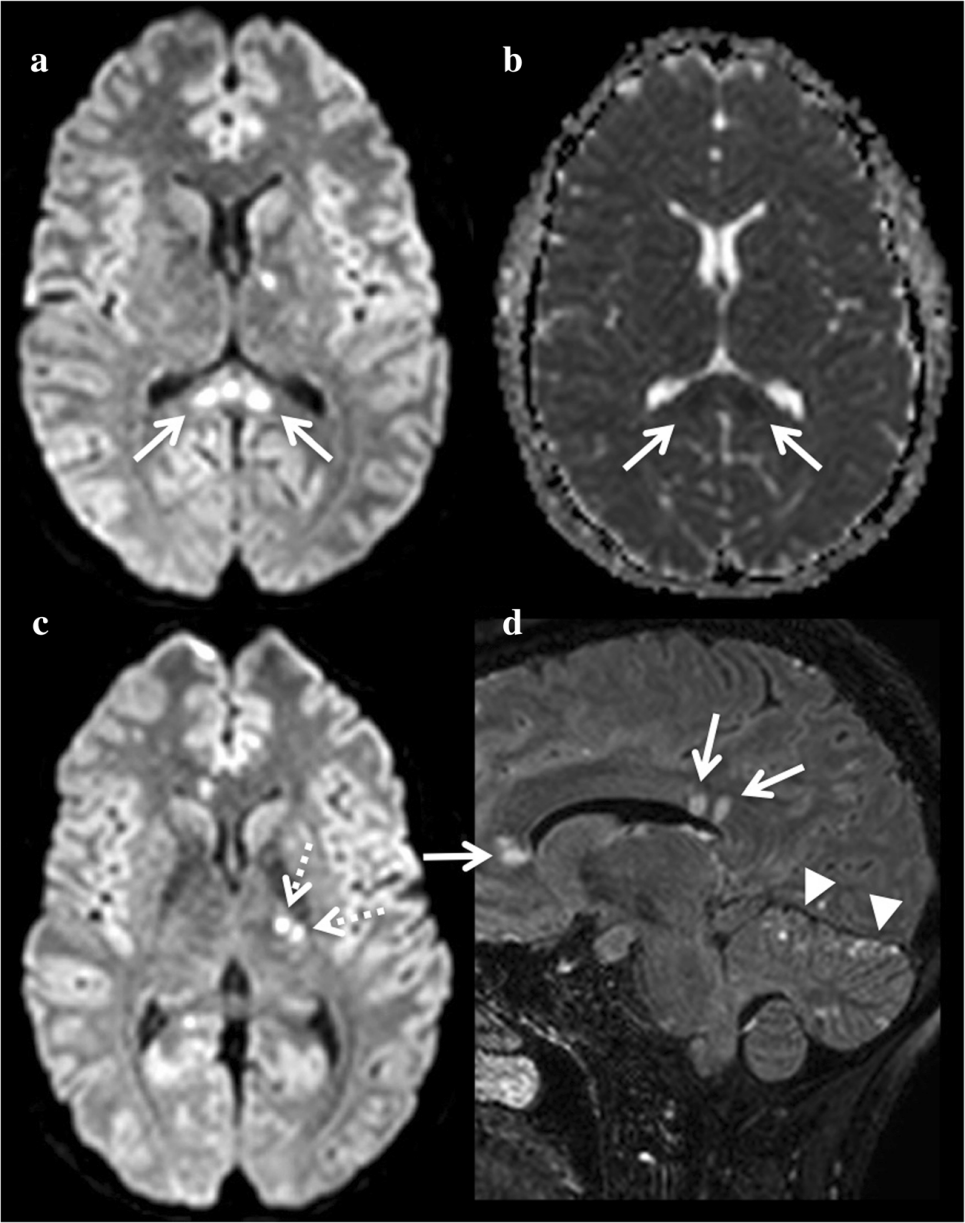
A 25-year-old woman with Susac’s syndrome presenting with vertigo and vertical diplopia. DWI shows restrictive lesions in the centre of the corpus callosum described as ‘snowballs’ (a, b, arrows) and in the posterior limb of internal capsule appearing as a ‘string of pearls’ (c, dotted arrows). Contrast-enhanced FLAIR shows small round white matter lesions (‘snowballs’) in the central fibres of the corpus callosum (d, arrows) and leptomeningeal contrast enhancement predominant in the posterior cranial fossa (d, arrowheads)
